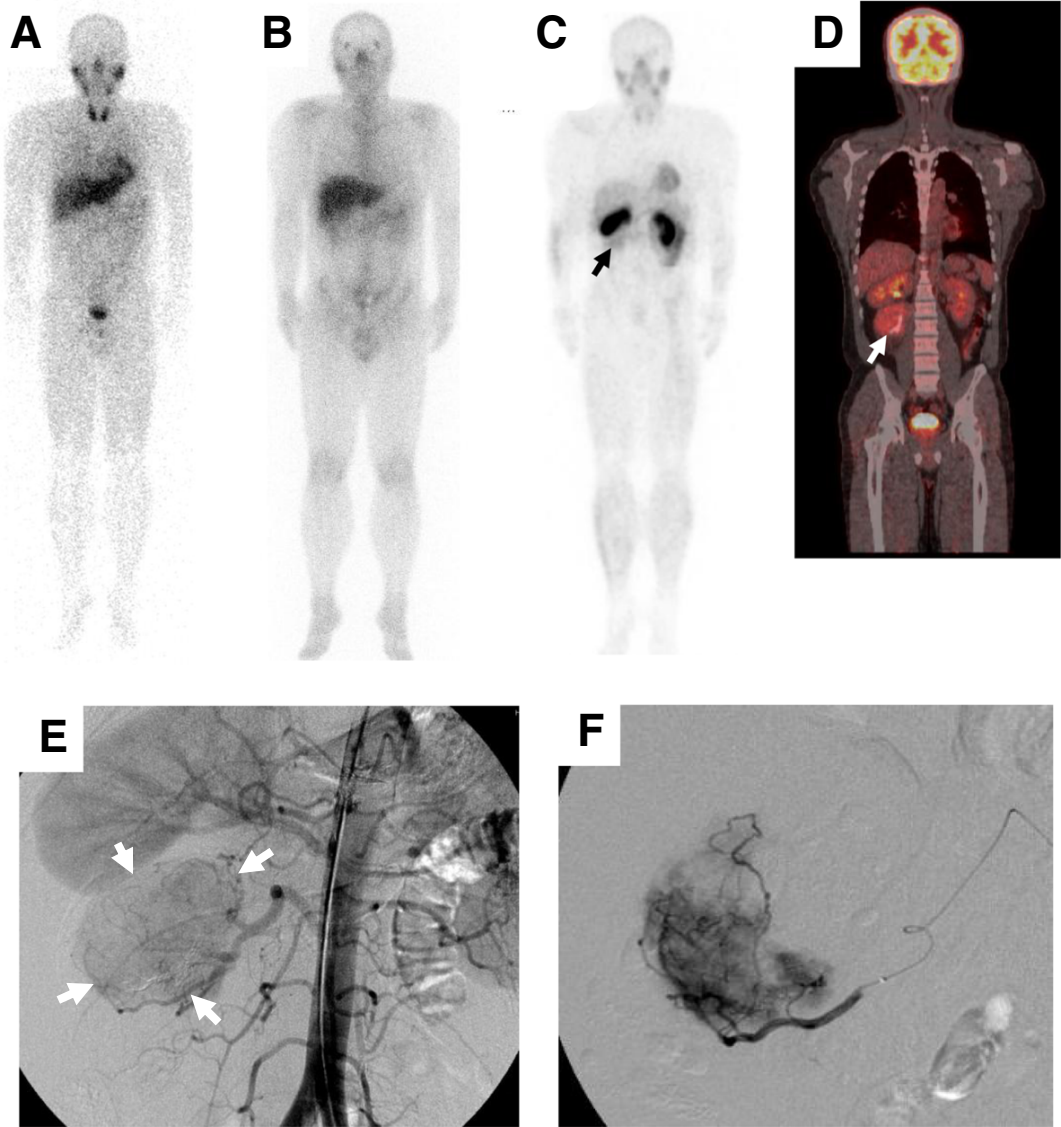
Radionuclide imaging and angiography. Both iodine-123 metaiodobenzylguanidine (A) and gallium-67 (B) scintigraphies were negative in the mass, whereas thallium-201 mildly accumulated in the tumor below the right kidney (C, arrow). Positron emission tomography was positive in the tumor at a standard uptake value of 4.7 without any other abnormal uptake in other parts of the body (D). Digital subtraction angiography revealed abundant vascularity of the tumor (E, arrow). Feeding vessels from the right lumbar arteries were embolized with porous gelatin particles (F).
